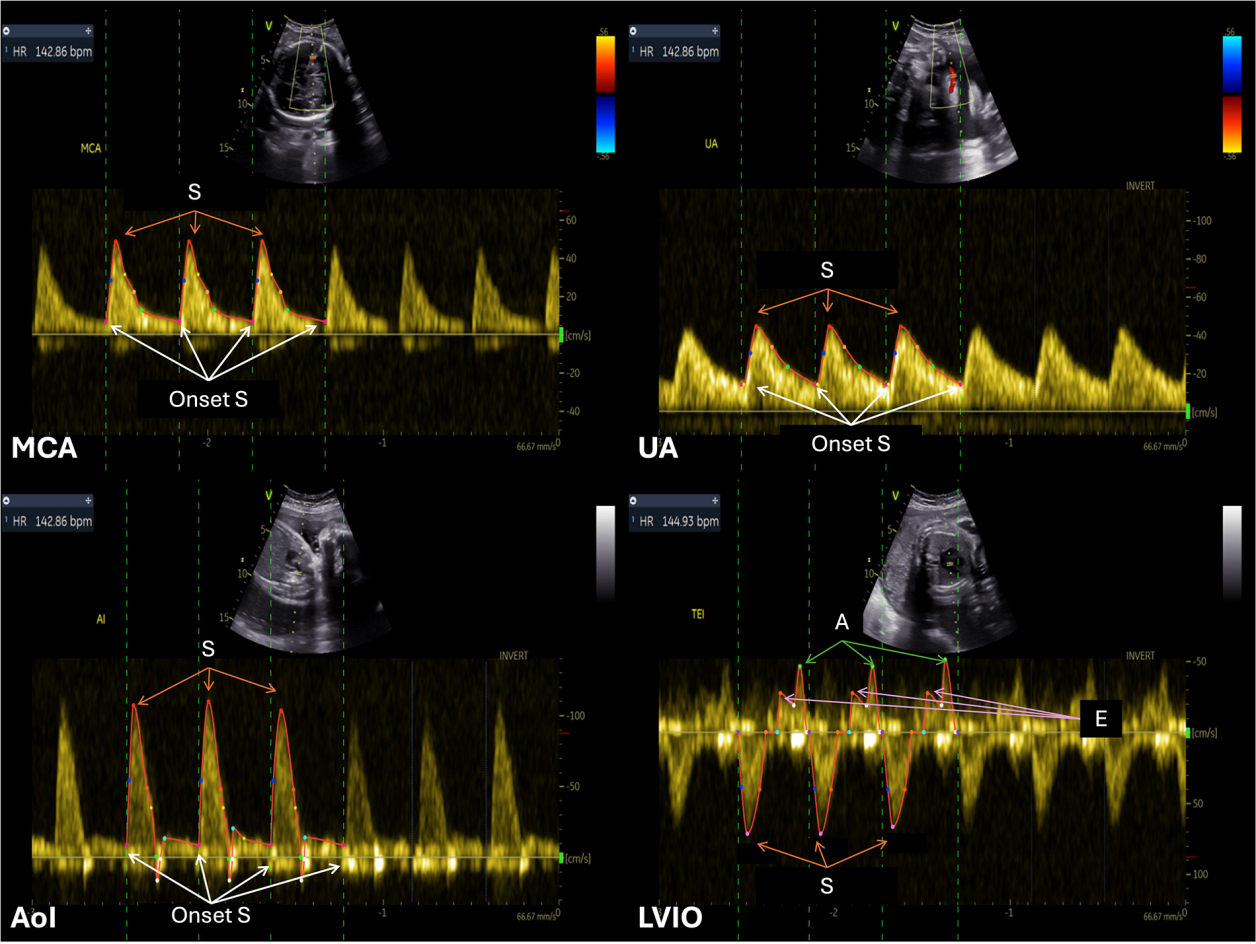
Example of annotated Doppler images from the feDoC dataset. The MCA, UA and AoI traces were delineated using the location of the S onset and the systolic peak value (S). The LVIO was traced with the S onset and systolic peak value to represent AV outflow and the E and A peaks were used for the MV inflow tracing.
